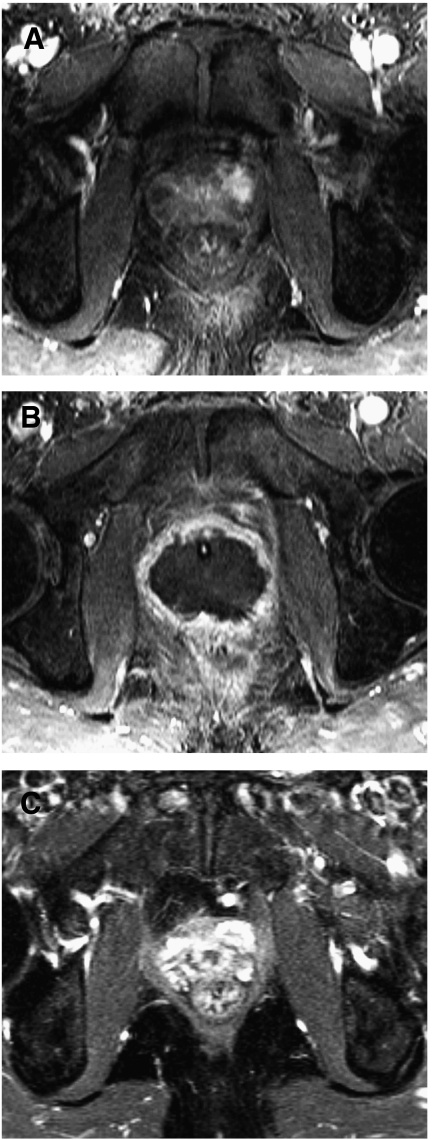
Contrast enhanced MRI changes in a successful treatment for prostate cancer using HIFU. (A) 1.5 Tesla dynamic contrast enhanced MRI using gadolinium prior to HIFU treatment demonstrating localised disease with a lesion in the left antero-lateral side of the gland (circled). (B) 1.5 Tesla dynamic contrast enhanced MRI using gadolinium at 2 weeks demonstrating poor perfusion in the prostate after HIFU treatment. Urethral catheter seen in-situ. (C) 1.5 Tesla dynamic contrast enhanced MRI using gadolinium at 6 months no residual prostate tissue and fibrotic reaction.
